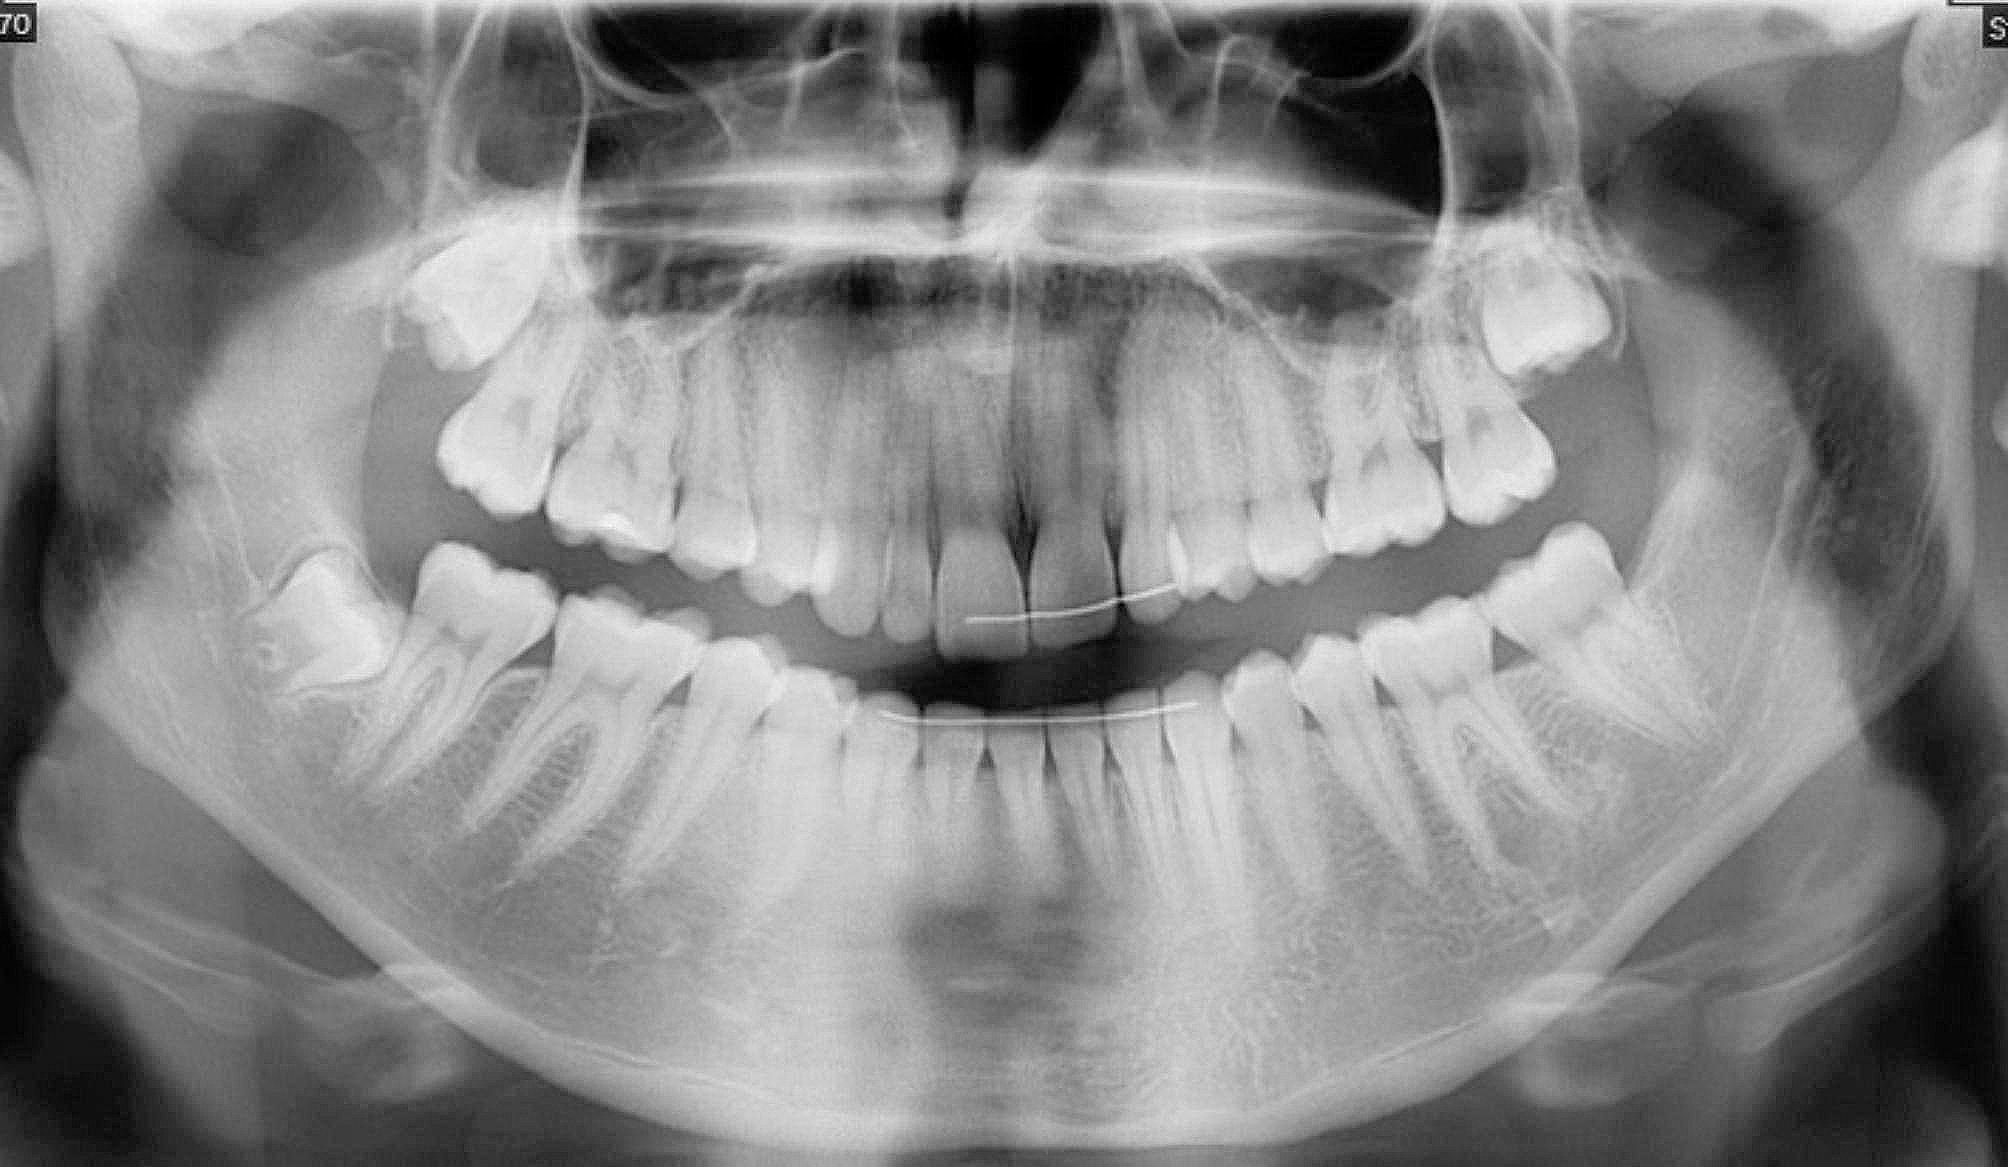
Panoramic X-ray at 6 months of follow-up. Legend: This view’s showing complete reossification of the bony defect around root of tooth #36
